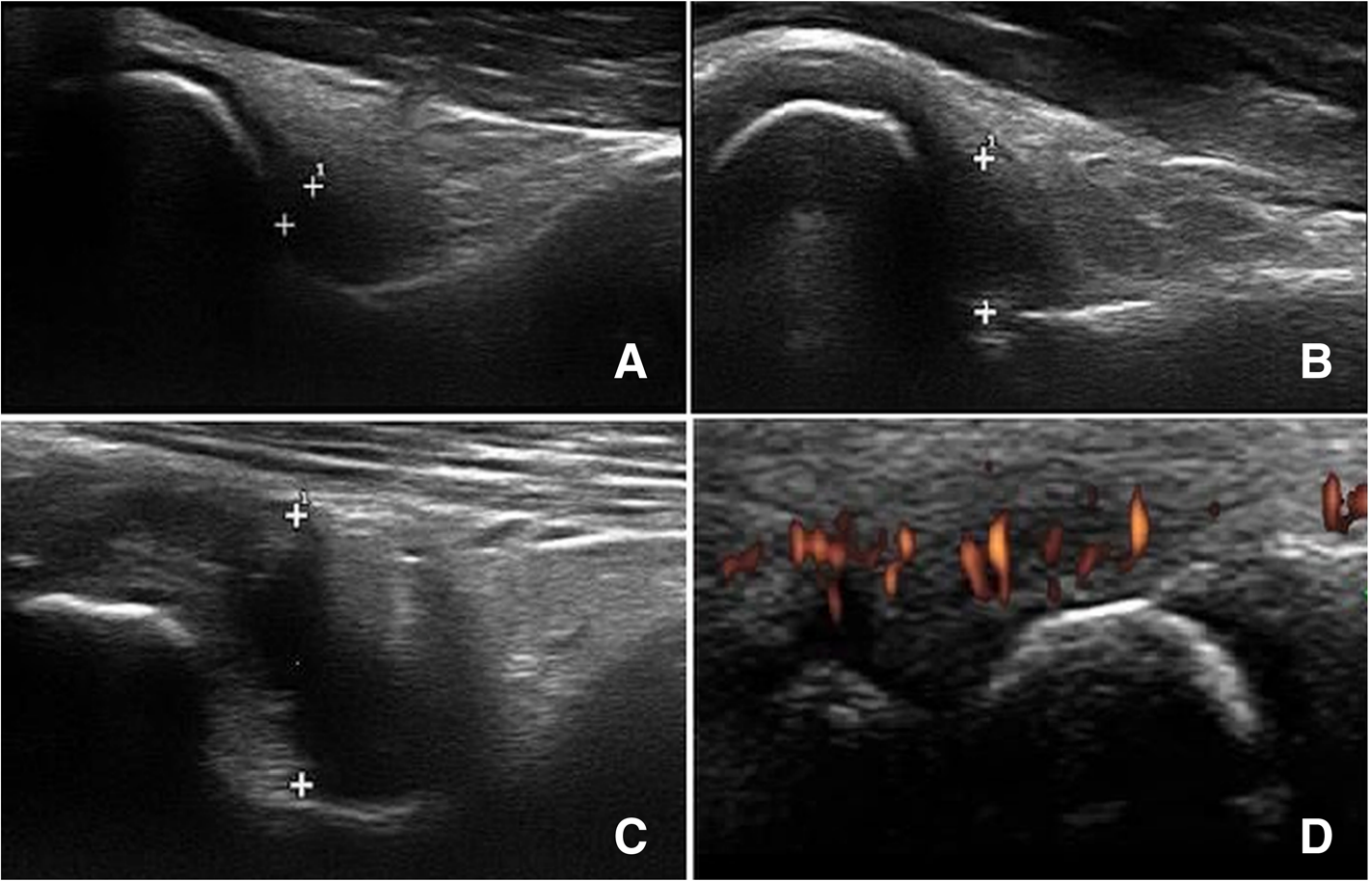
Ultrasonographic parameters. Synovitis in the anterior elbow (a. Grade 1; b. Grade 2; c. Grade 3) and synovial flow in the radiocarpal wrist (d. Positive power Doppler signal)
